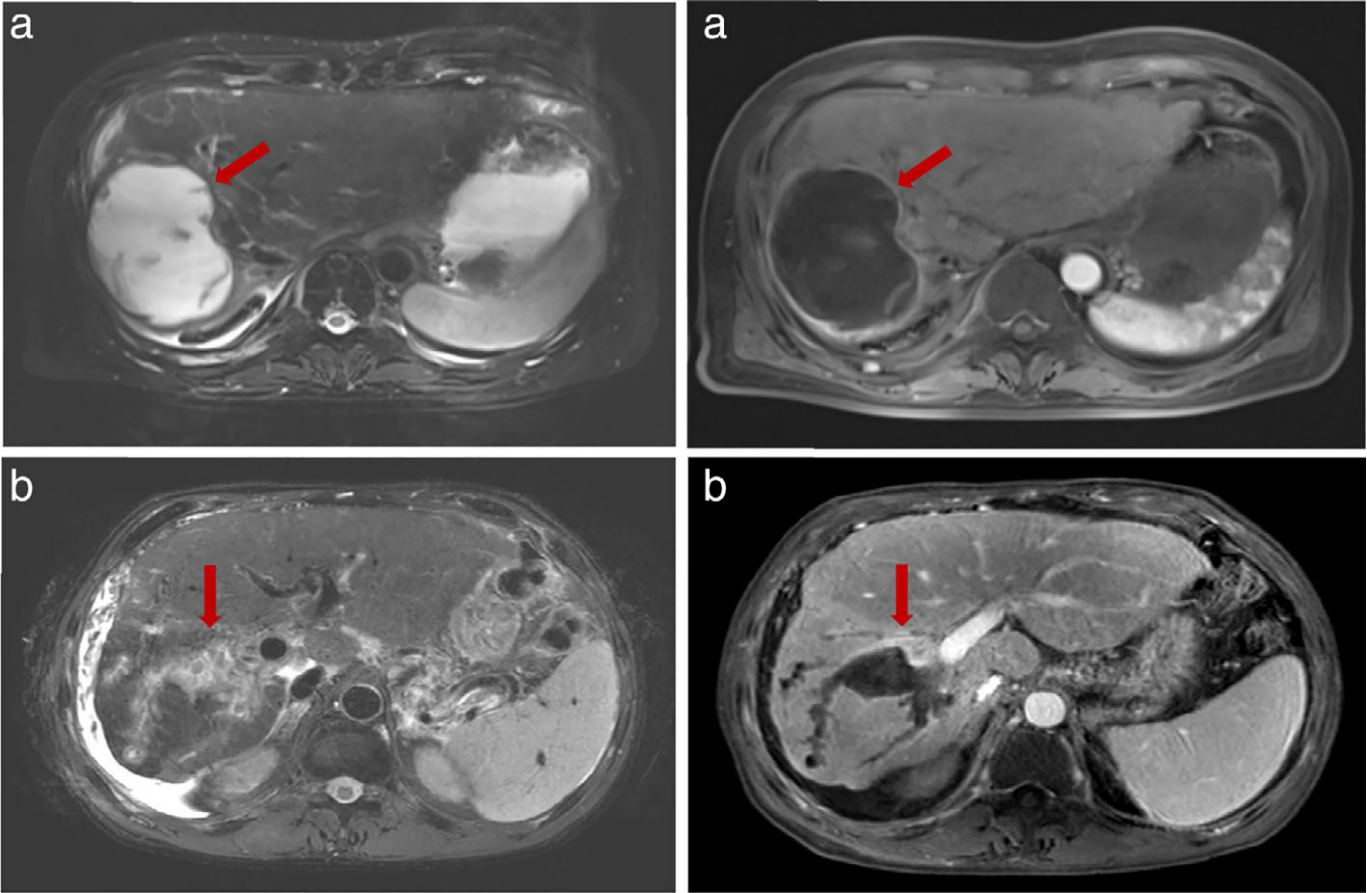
MRI image scans revealed biliary lesion in patients with bronchobiliary fistula. (a) Red arrow indicates the liver abscess after ablation. (b) Red arrow showed the biliary tract dilatation after ablation.
